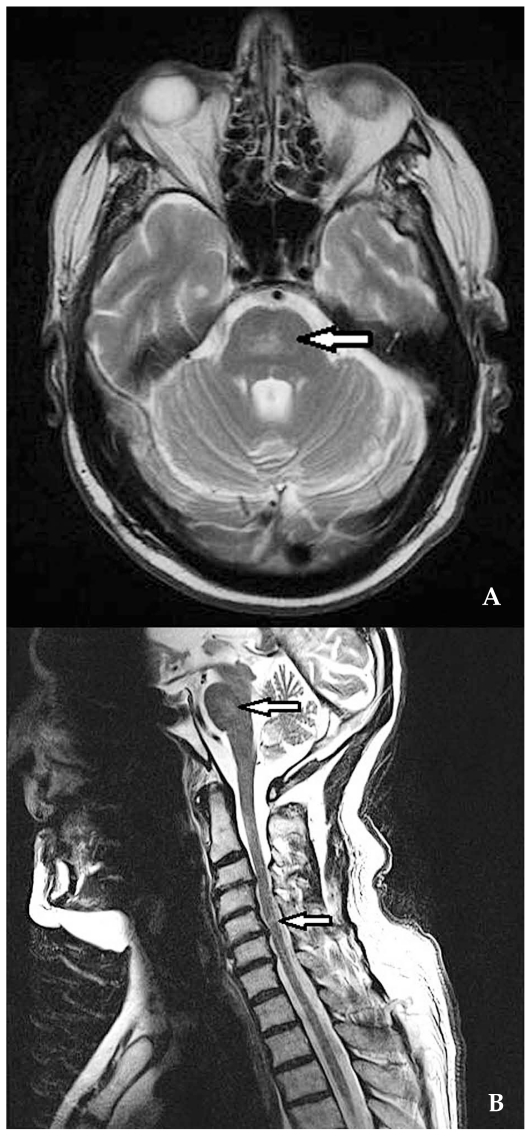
MRI in disseminated CNS vasculitis (T2-hyperintense lesions) with localizations, in the same patient, both in the central pontine area (A) and in the cervical cord (B).
